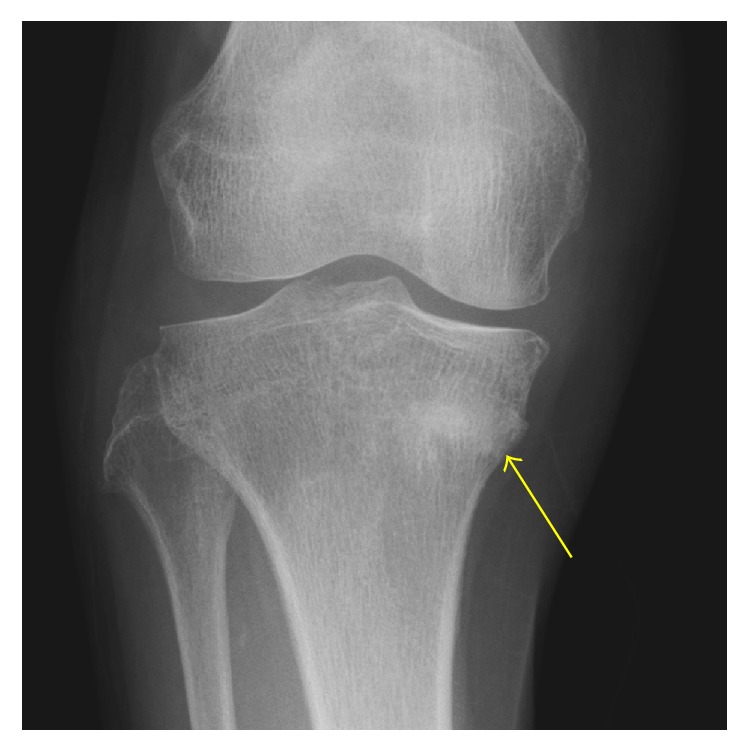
The anteroposterior radiograph of right knee 4 months after the onset showing the bone union of the insufficiency fracture (yellow arrow).
